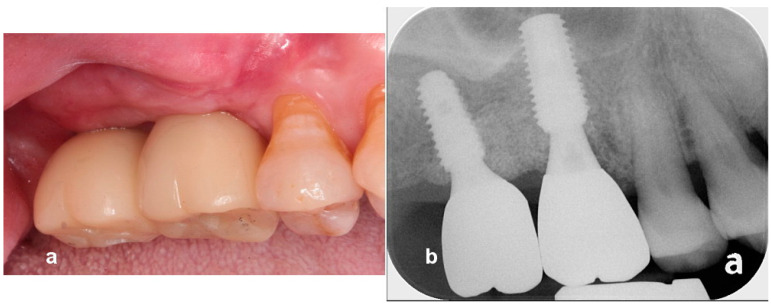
(a) An intraoral image after 6 months of loading reveals the attached keratinized gingiva around the zirconia-based final restoration. (b) A periapical radiograph after 6 months of loading indicates a stable augmented ridge over implant platforms.
